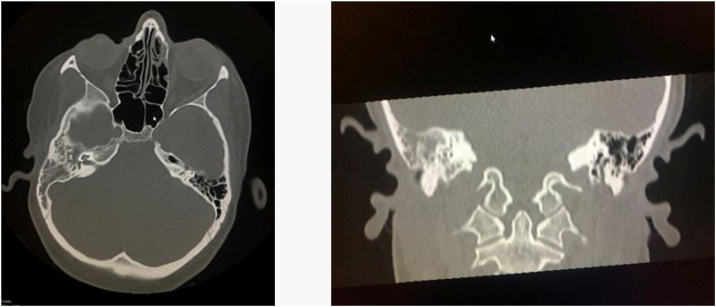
CT-scan of temporal bone demonstrating a hypointensity tissue filling all the middle ear and epytimpanic space.
